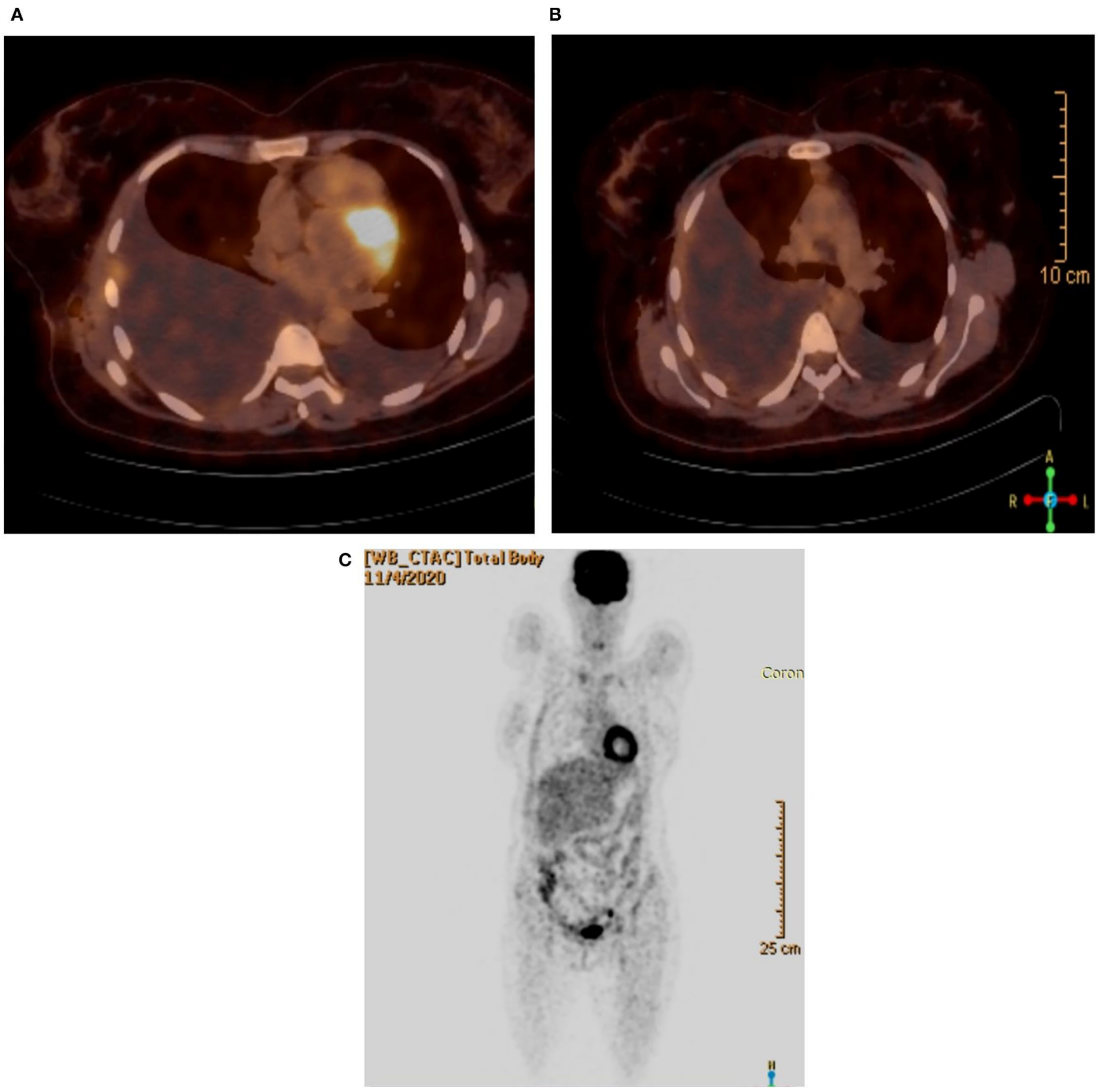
(A) PET/CT scan showing the lungs. (B) PET/CT scan without hypermetabolic activity. (C) Coronal view.
